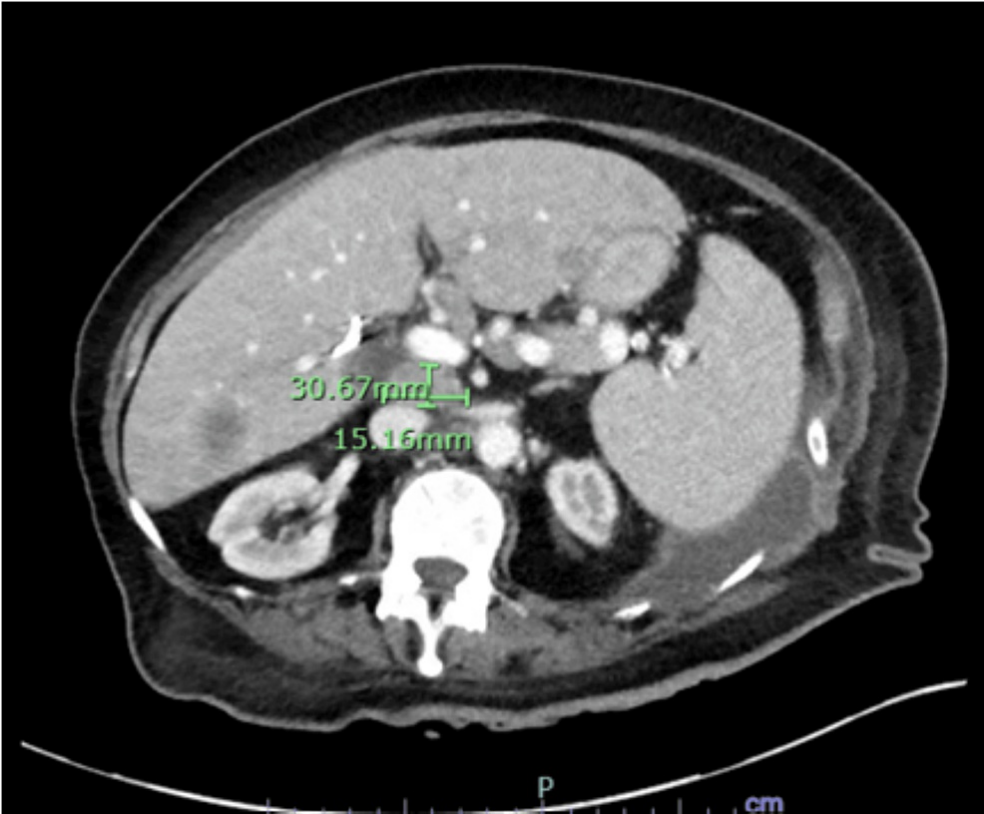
Abdominal CT showing portacaval and aortocaval lymph nodes
